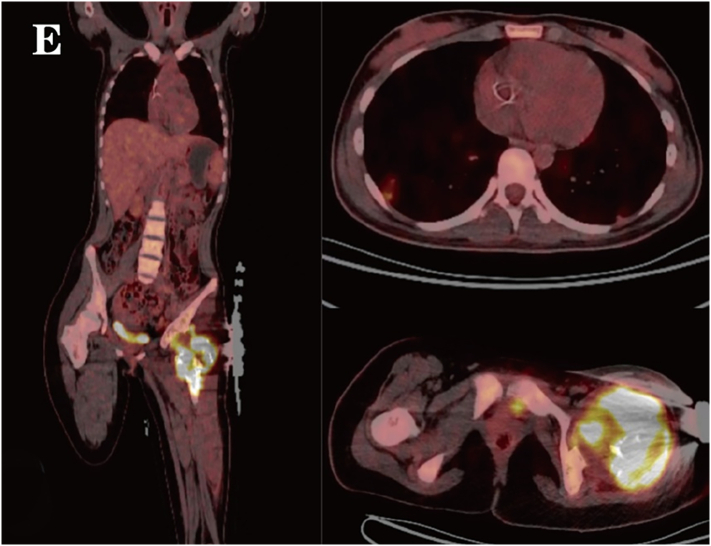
PET-CT revealed hypermetabolic activity in the soft tissue mass at the proximal left femur with cortical destruction, suggesting tumor invasion. Multiple hypermetabolic nodules in both lungs indicated metastasis.
